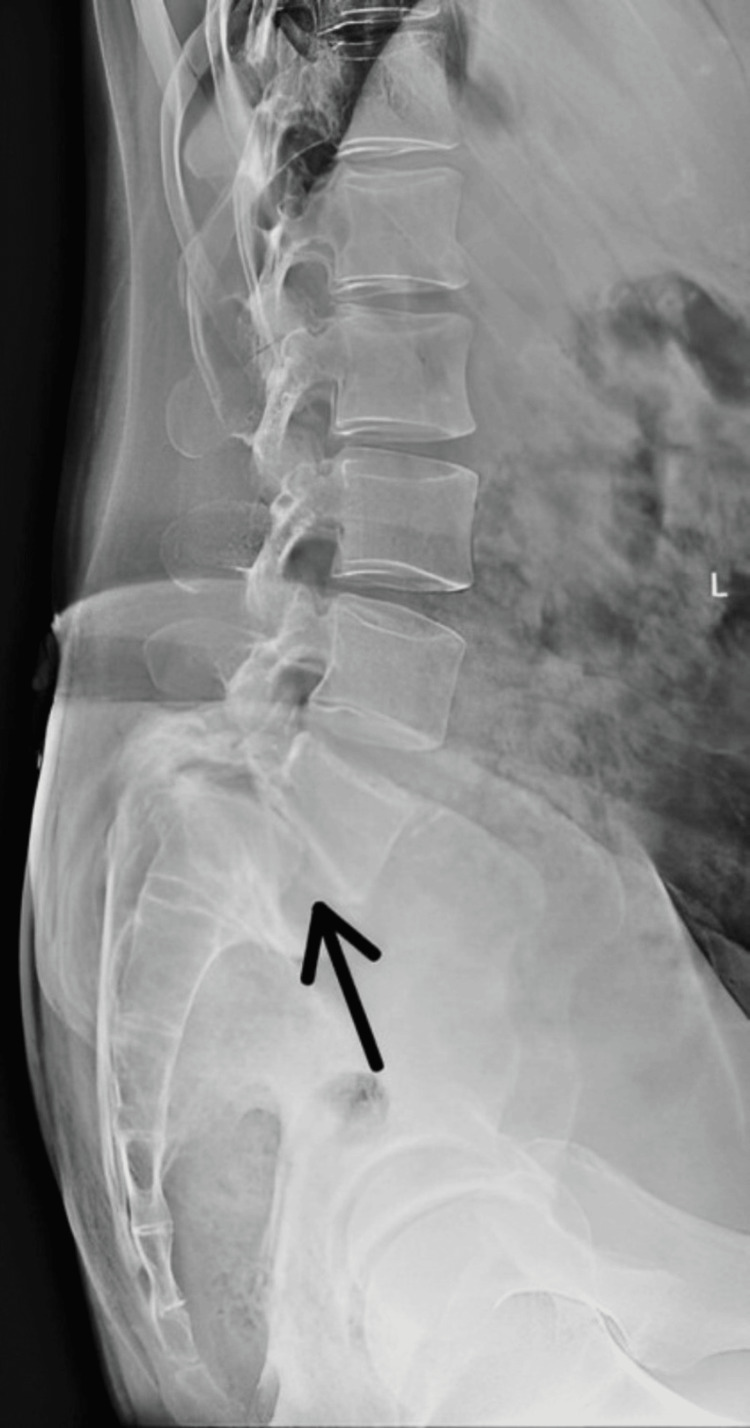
Lumbar spine X-ray The black arrow indicates L5-S1 anterolisthesis
